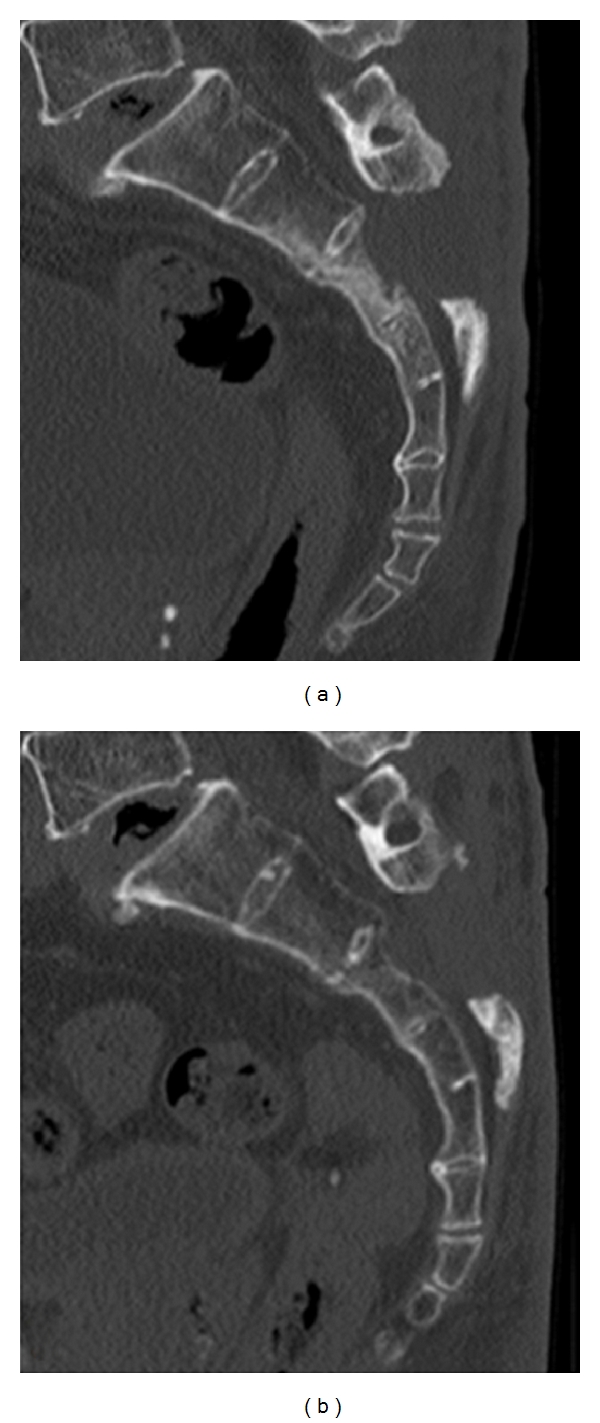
Postoperative follow-up reconstructed CT. (a) Two months after surgery, the third sacral vertebral body showed a high-density area. (b) One year after surgery, the third sacral vertebral body had jointed without displacement.
